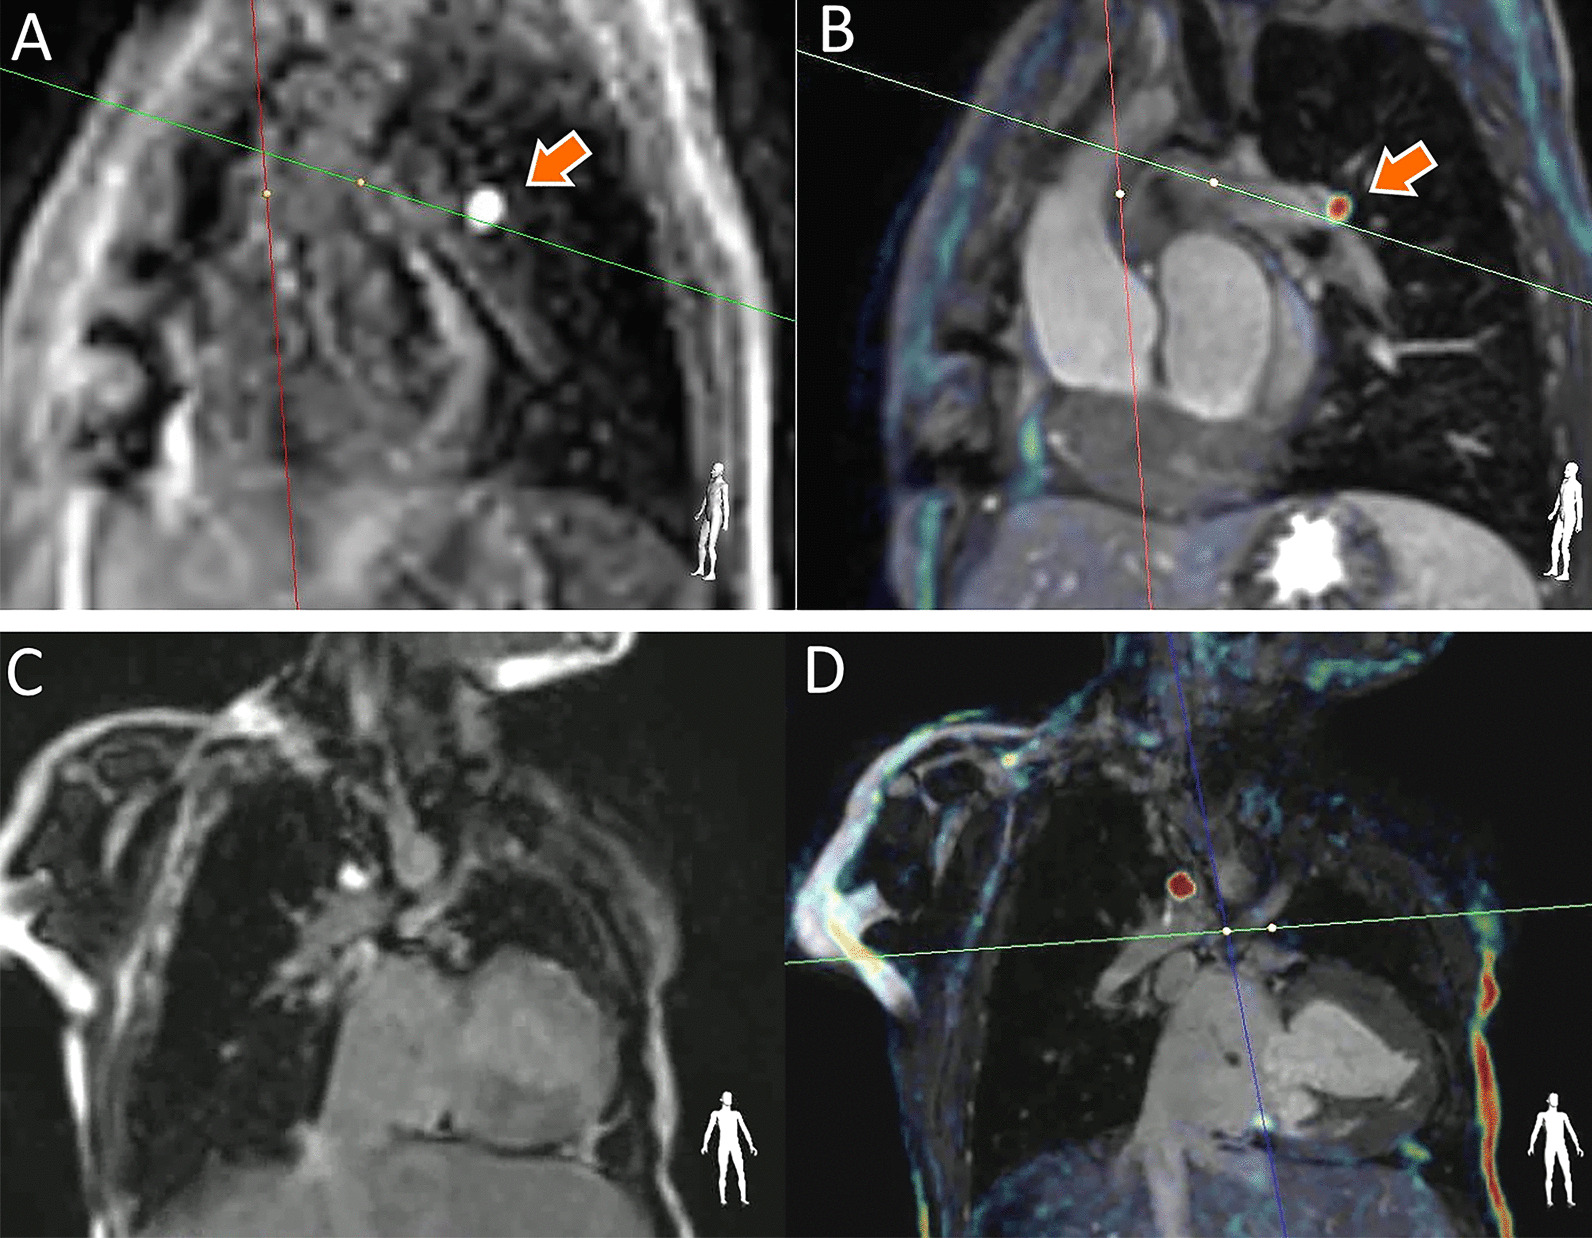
A, B A gadolinium-filled catheter in the left pulmonary artery of another patient. A The proposed real-time T1-weighted spoiled gradient echo image containing bright, isolated gadolinium filled balloon signal (orange arrow) with suppressed anatomical detail. B The real-time image from (A) with a manually-selected threshold applied to overlay the brightest (isolated catheter) signal on the high-resolution 3D dataset acquired at the beginning of the session. This provides the interventionalist with both high-resolution anatomical detail and clear visualization of the gadolinium- filled balloon wedge catheter tip. C, D The catheter in the Glenn anastomosis of a different patient using the bSSFP pSAT acquisition (C), and the proposed T1-overlay technique (D) demonstrating improved visualization of the catheter tip and anatomy. This improved catheter tracking can be further appreciated in Additional file 1, which shows the real-time T1-weighted images of the catheter in another patient during a right heart catheterization, and the resulting iSuite overlay that is projected into the scan room to guide the interventionalist
